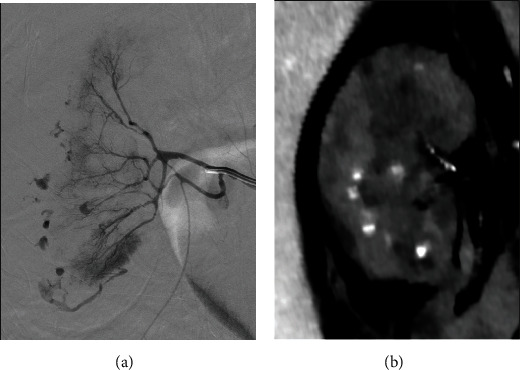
Digital subtraction angiography of the right renal artery (a) and coronal venous phase CT image (b) demonstrate active extravasation from the subsegmental arteries.
